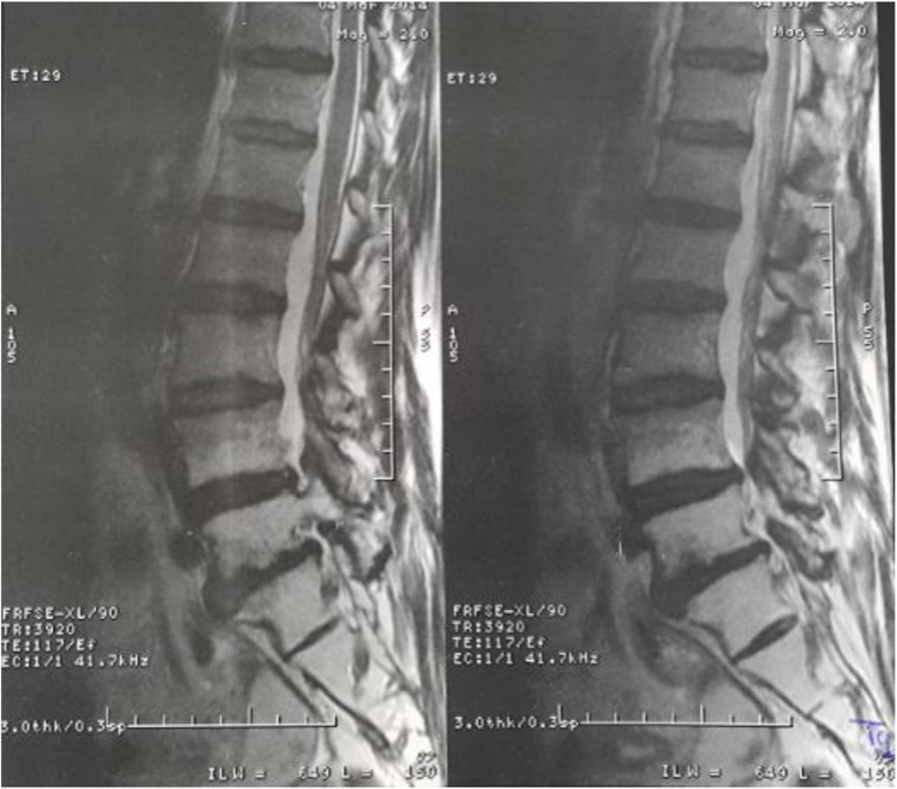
Spinal MRI T2-weighted sequence
